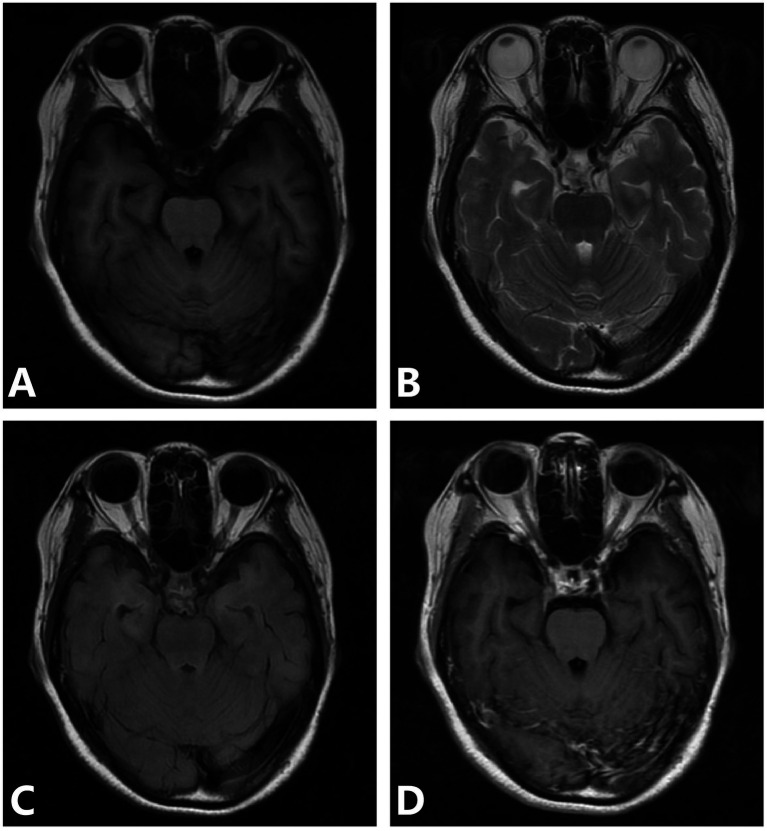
(A–D) Parenchymal type neurosyphilis, a 56-year-old female presented with sudden onset of unresponsiveness and difficulty in speaking for 1 day. MRI showed bilateral temporal lobe and hippocampal atrophy, increased signal intensity on T2WI and FLAIR, and no enhancement on enhanced scan. Panel (A) (T1WI): shows bilateral temporal lobe atrophy; panel (B) (T2WI): displays increased signal intensity in the hippocampal region; panel (C) (FLAIR): demonstrates hyperintense lesions with atrophy; panel (D) (T1WI-CE): shows no significant enhancement.
